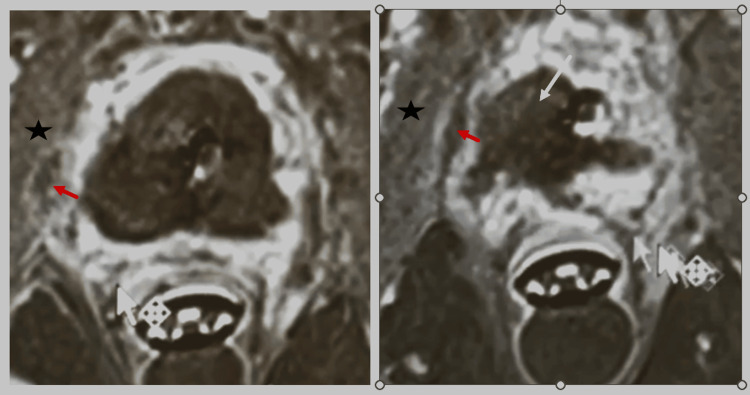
Post-ablation contrast-enhanced axial T1-weighted MRI Post-treatment contrast-enhanced axial T1-weighted images following transurethral ultrasound ablation (TULSA). The left panel demonstrates the expected treatment effect involving the obturator internus muscle superior to the ablation zone (star; red arrow). The right panel demonstrates the absence of contrast enhancement within the treated apical lesion (white arrow), consistent with successful ablation, with adjacent treatment effect involving the ipsilateral levator ani and obturator internus musculature (star; red arrow).
